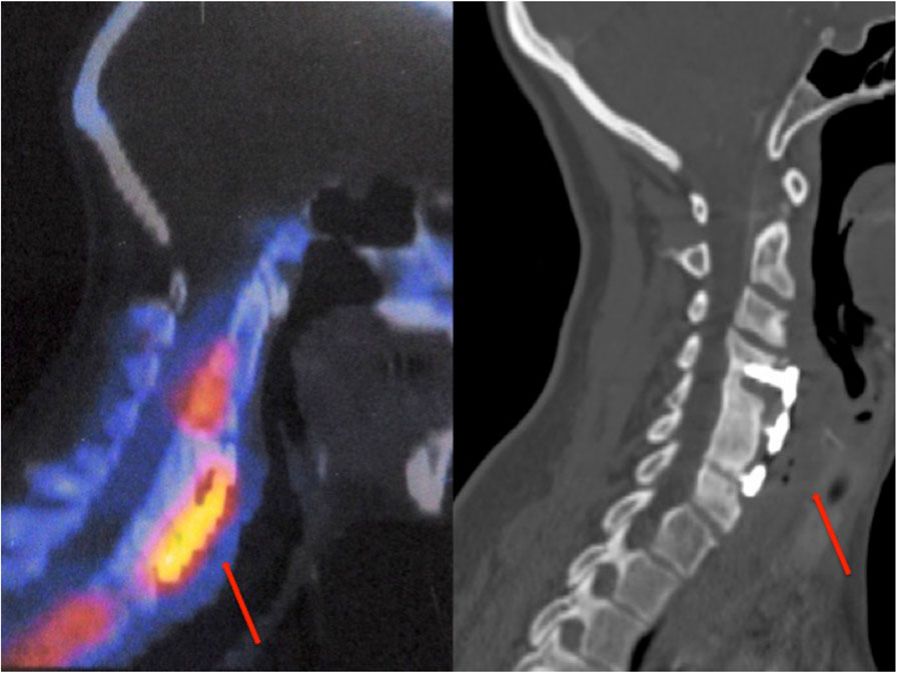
Single-photon emission computed tomography shows spondylitis in the cervical spine (C4-C6) and an inflammatory process in the nasopharynx with free air in subcutaneous tissue and increase in the prevertebral space of > 2 cm (*red arrow*)
